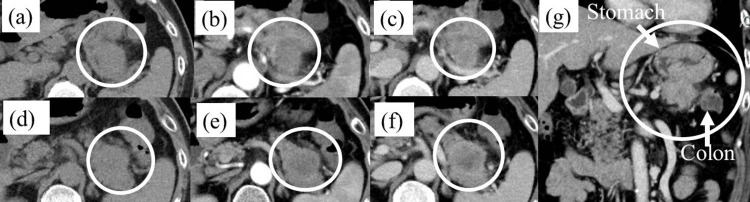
Abdominal contrast-enhanced computed tomography (CT) one month after the first visit to our department (a and d) Plain; (b and e) arterial phase; (c, f, and g) portal phase; (a-f) axial section; (g) coronal section. Abdominal enhanced CT image showing a 35-mm-in-diameter hypovascular enlarged lesion in the pancreatic tail in contact with the stomach ((a-c and g), circled area) and adjacent to the transverse colon ((d-g), circled area).
